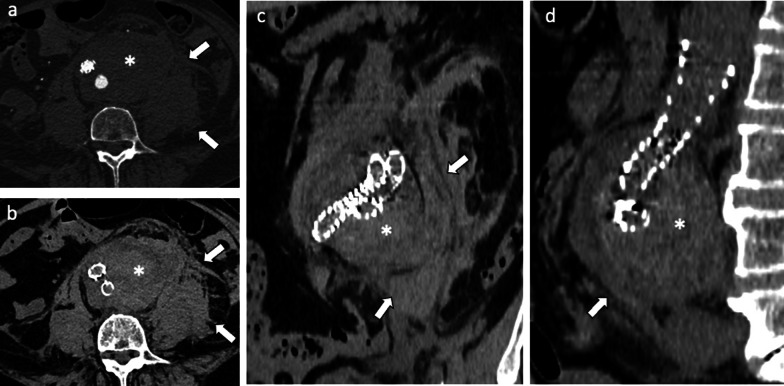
65-year-old man with *aneurismatic sac rupture*: Axial CT images on (a) pre-contrast, (b) arterial phases and (c) coronal and (d) sagittal arterial phase show left antero-lateral rupture of the aneurysm sac with periaortic hematoma (*) and blood effusion close to the left psoas muscle
